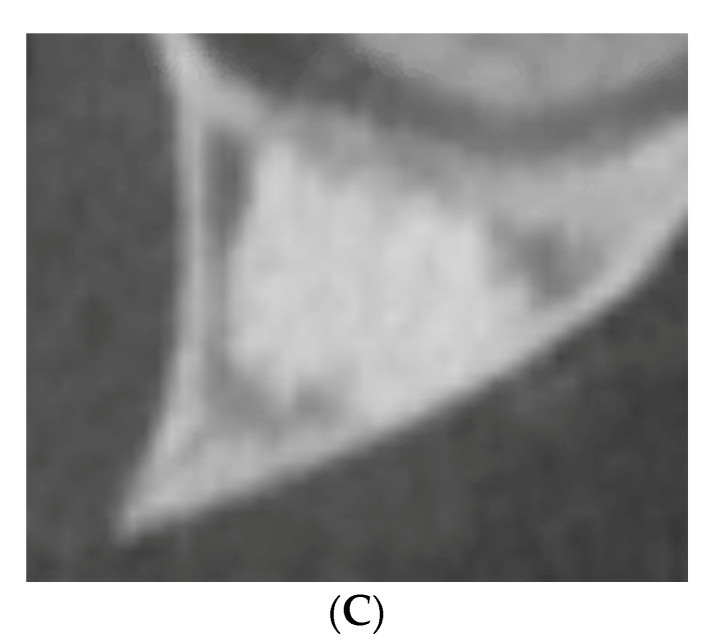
(A–C) Case 1. Pelvic axial CT shows an enlarged lesion 5 years after original discovery, (A) Initial CT displays a 16 mm sclerotic focus in the left posterior acetabulum. (B) Repeat CT demonstrates growth of the bone lesion to 21 mm, central radiodensity and peripheral “rose thorn” appearance. (C) Higher magnification emphasizes characteristic features. Despite its enlargement, it was felt to be otherwise typical for BI, so observation was recommended.
